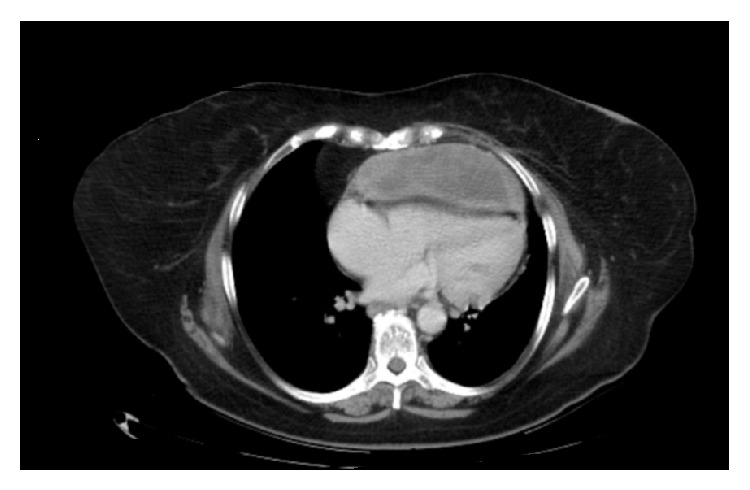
CT of chest showing 9.9 × 11.5 × 14.2 cm heterogeneous mass, with internal necrosis and calcification, associated with the anterior pericardium and displacing the heart superiorly and posteriorly.
